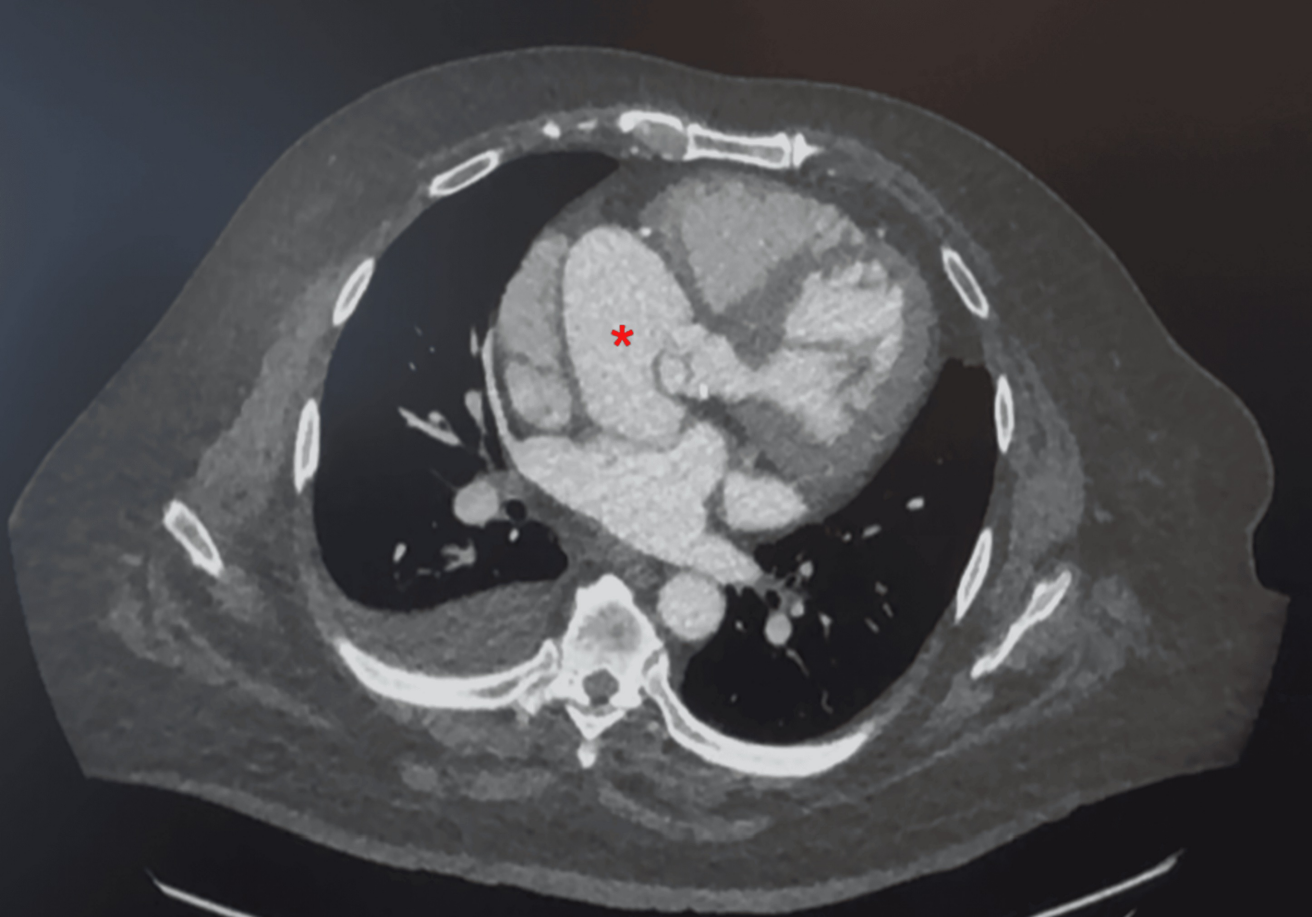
CT angiography thorax showed a large aortic root abscess/pseudoaneurysm, measuring 6.2 cm × 4.4 cm × 5.6 cm and the neck of the pseudoaneurysm measured 17 × 13 mm (indicated by the red *).
